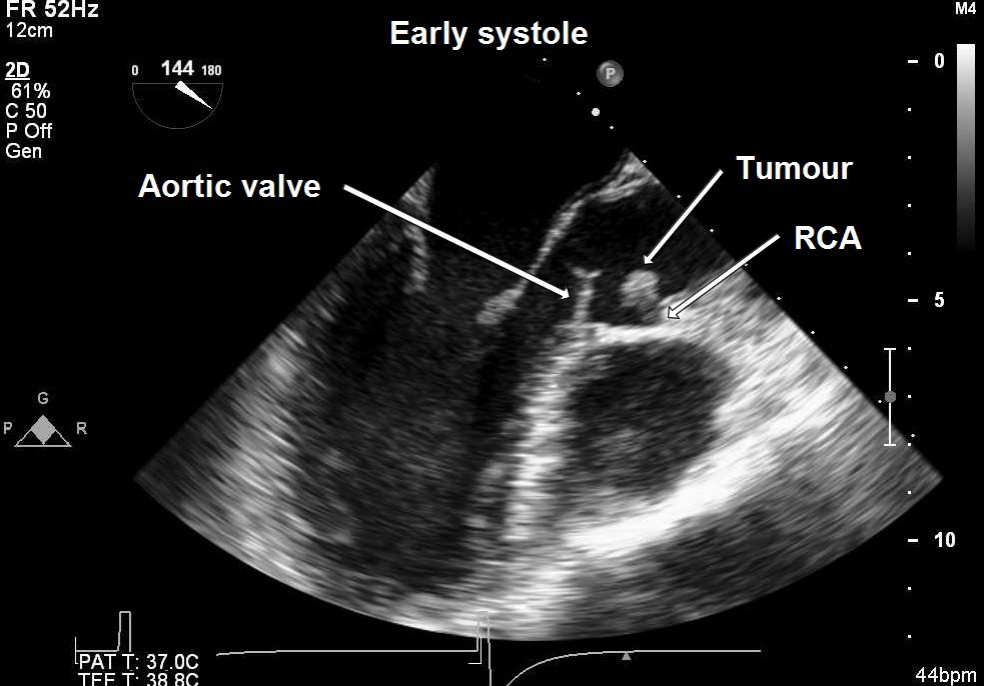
Trans-oesophageal echocardiography showing supra valvular tumour during early systole moving away from the right coronary artery (RCA) ostium (ME AV long-axis view).
